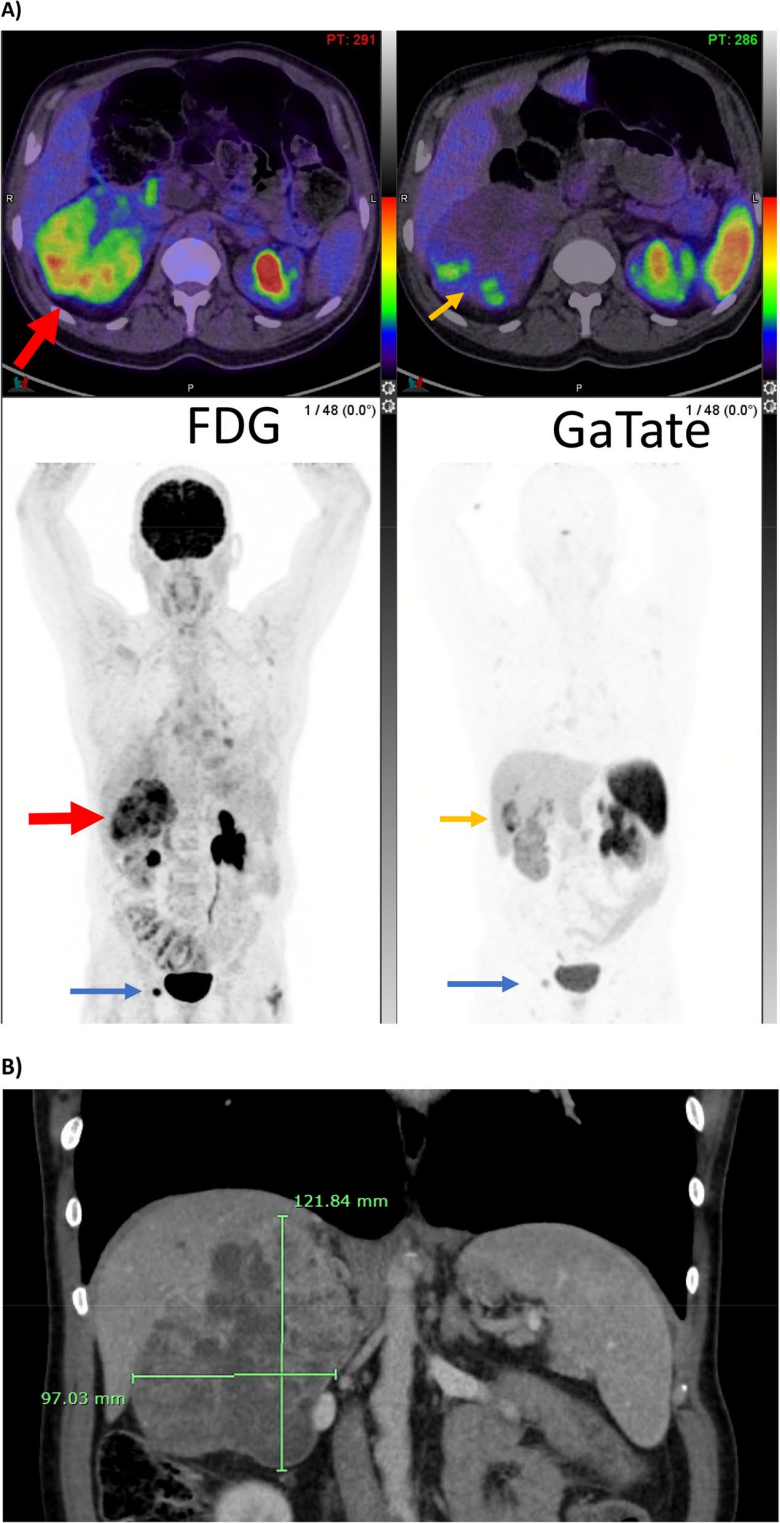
A FDG (left panel) and GaTate (right panel) maximum intensity projections (bottom) and upper abdominal axial slice demonstrating a moderate to intensely FDG-avid right adrenal lesion (red arrows) with corresponding patchy, peripheral somatostatin receptor expression on GaTate (orange arrows), as well as a single intensely FDG-avid right inguinal lymph node (blue arrows). B Contrast adrenal CT demonstrating measurements of the large right adrenal mass (rulers)
